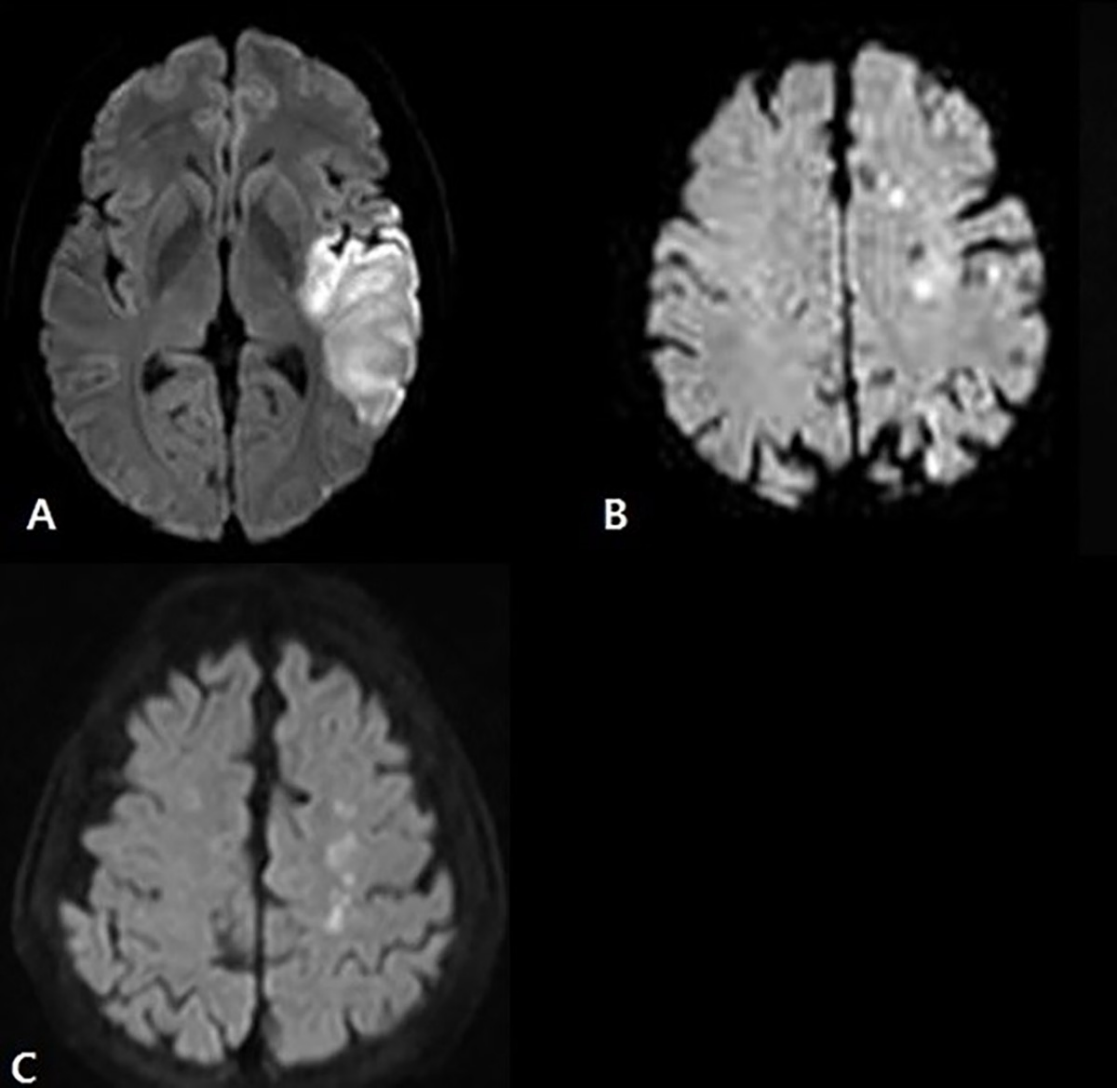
Three infarct patterns on diffusion-weighted magnetic resonance images. A. Large territorial lesion. B. Disseminated small lesions. C. Border zone infarction.
